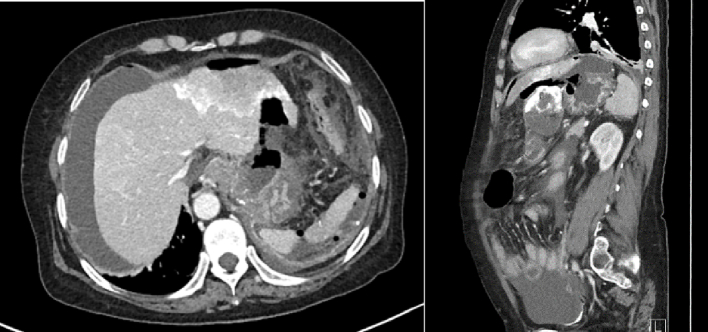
CT of the abdomen shows gastric-wall perforation and a previously concealed rHCC at the left hepatic lobe.
